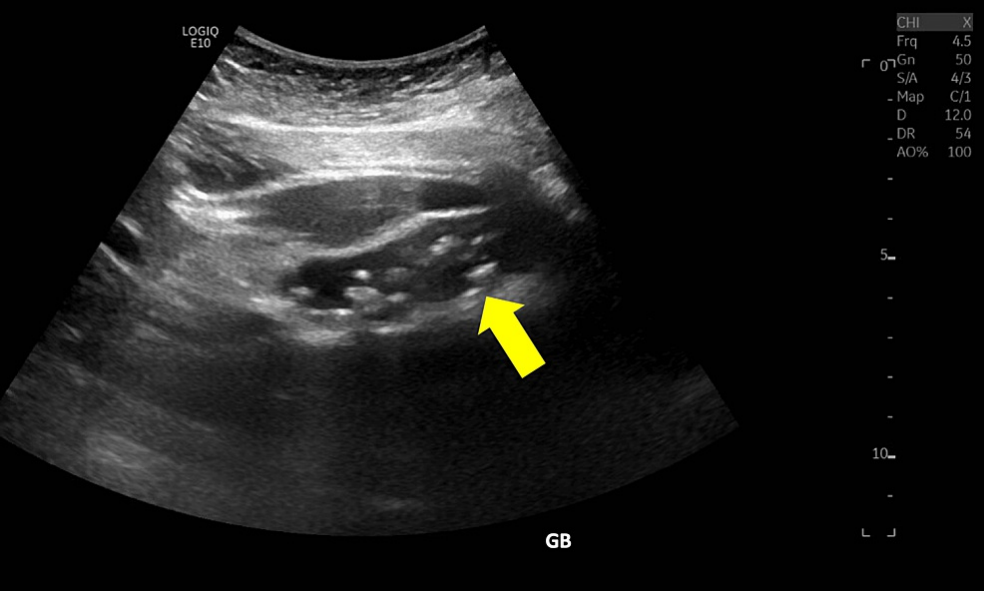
Ultrasound image of the left upper quadrant showing multiple calculi (yellow arrow) within the gallbladder
